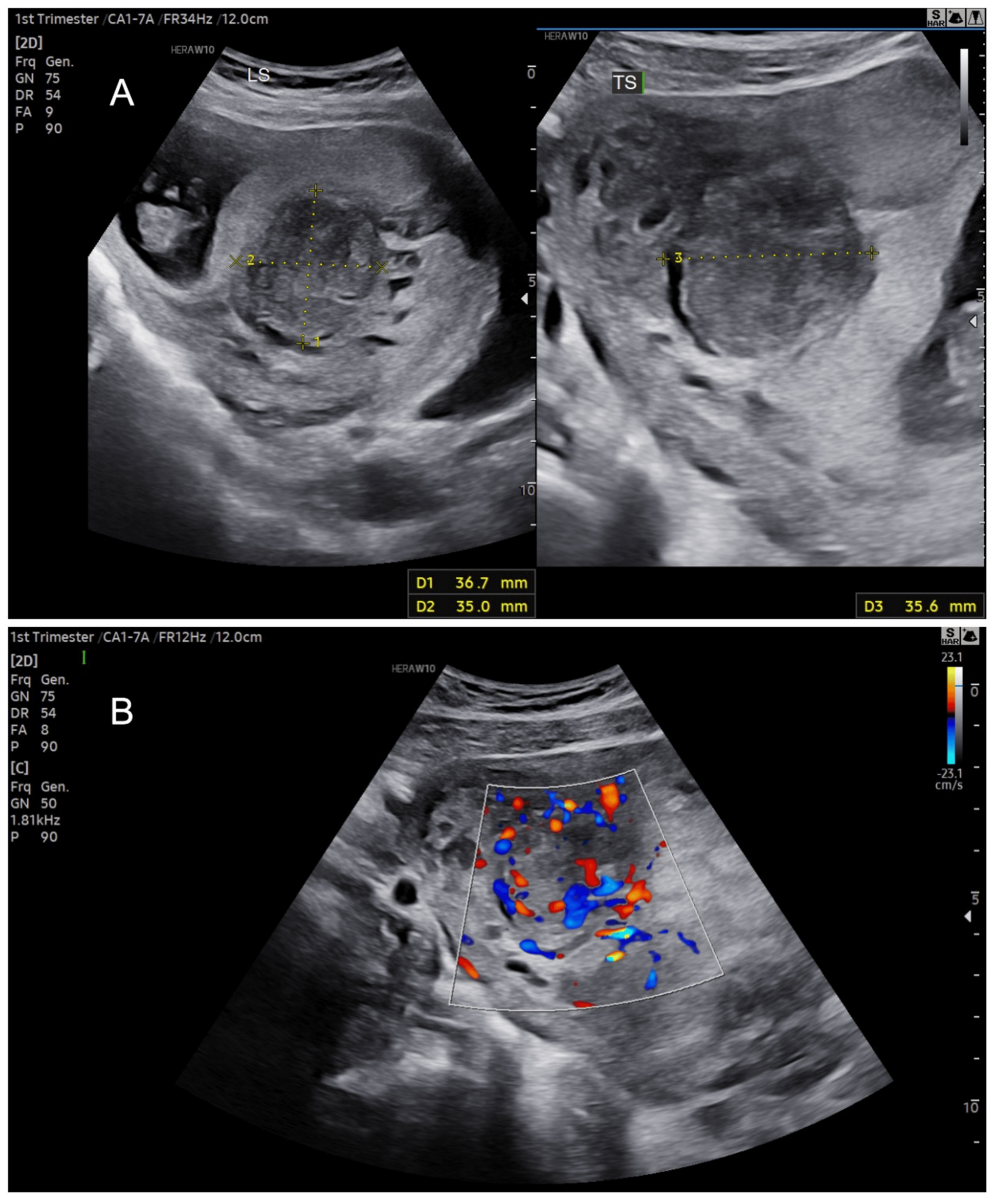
Ultrasound at nine weeks of gestation showing (A) the uterine mass and (B) high vascularity on Doppler examination
